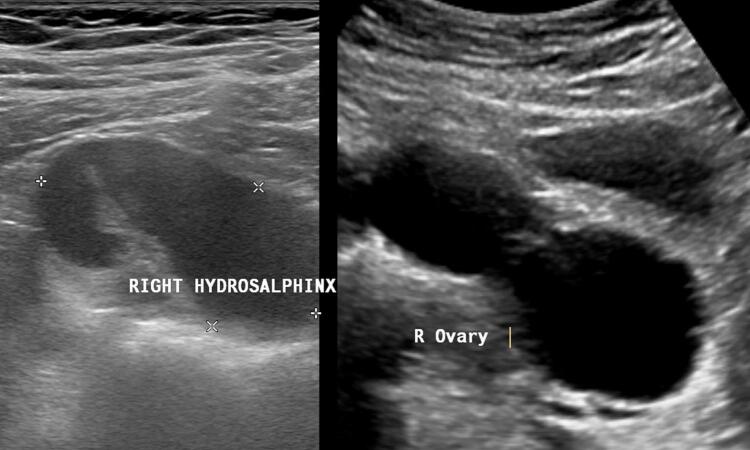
Transabdominal ultrasonography showing large tubular dilated cystic lesion in right adnexa separate from right ovary suggestive of severe right hydrosalpinx.
